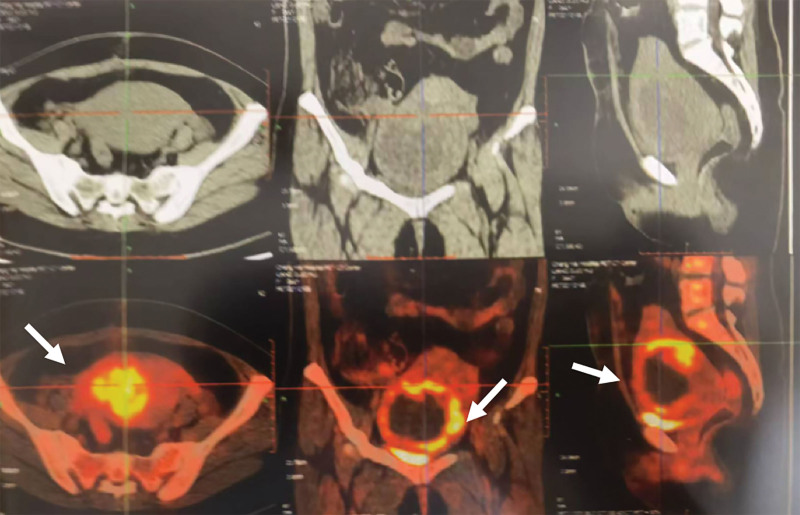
PET-CT scan showed an irregular mass in the anterior wall of the uterine, along with surrounding intensive ^18^F-fluorodeoxyglucose uptake (SUVmax of 5.8), without enlarged lymph nodes.
